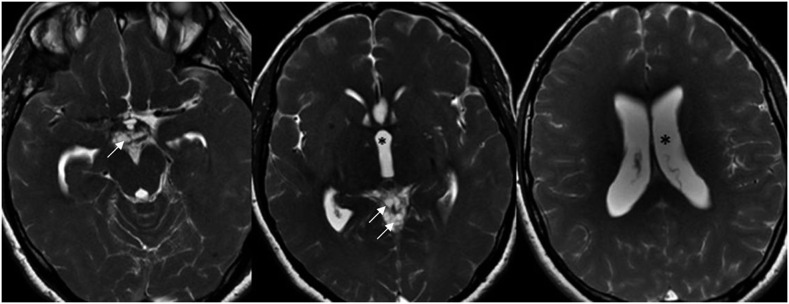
Brain MRI volumetric T2-weighted images. Hydrocephalus (asterisk) secondary to multiple cysts involving the suprasellar region and the supravermian cistern (arrows).
